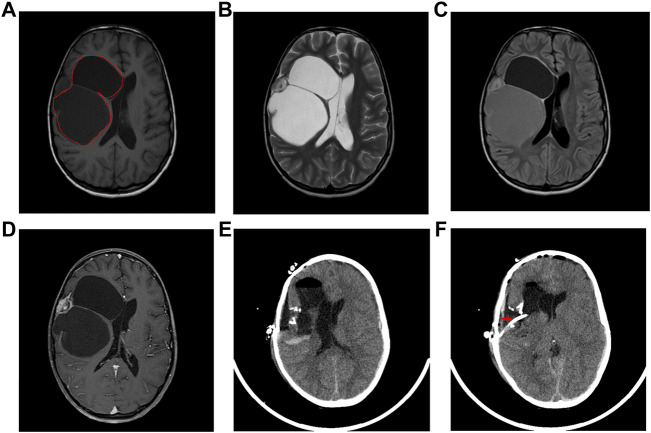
Radiological features of the tumor. (A) The tumor region is demarcated using red lines in an Axial T-1 weighted image, (B) T-2 weighted, (C) Flair and (D) T-1 contrast MRI showing a multiloculated tumor with solid and cystic areas causing mass effect on the ventricle with midline shift. (E) Post-operative axial CT scan showing tumor excision and decreased mass effect and return of midline structures. (F) Axial CT scan showing the catheter (marked by a red arrow) in the cystic cavity.
